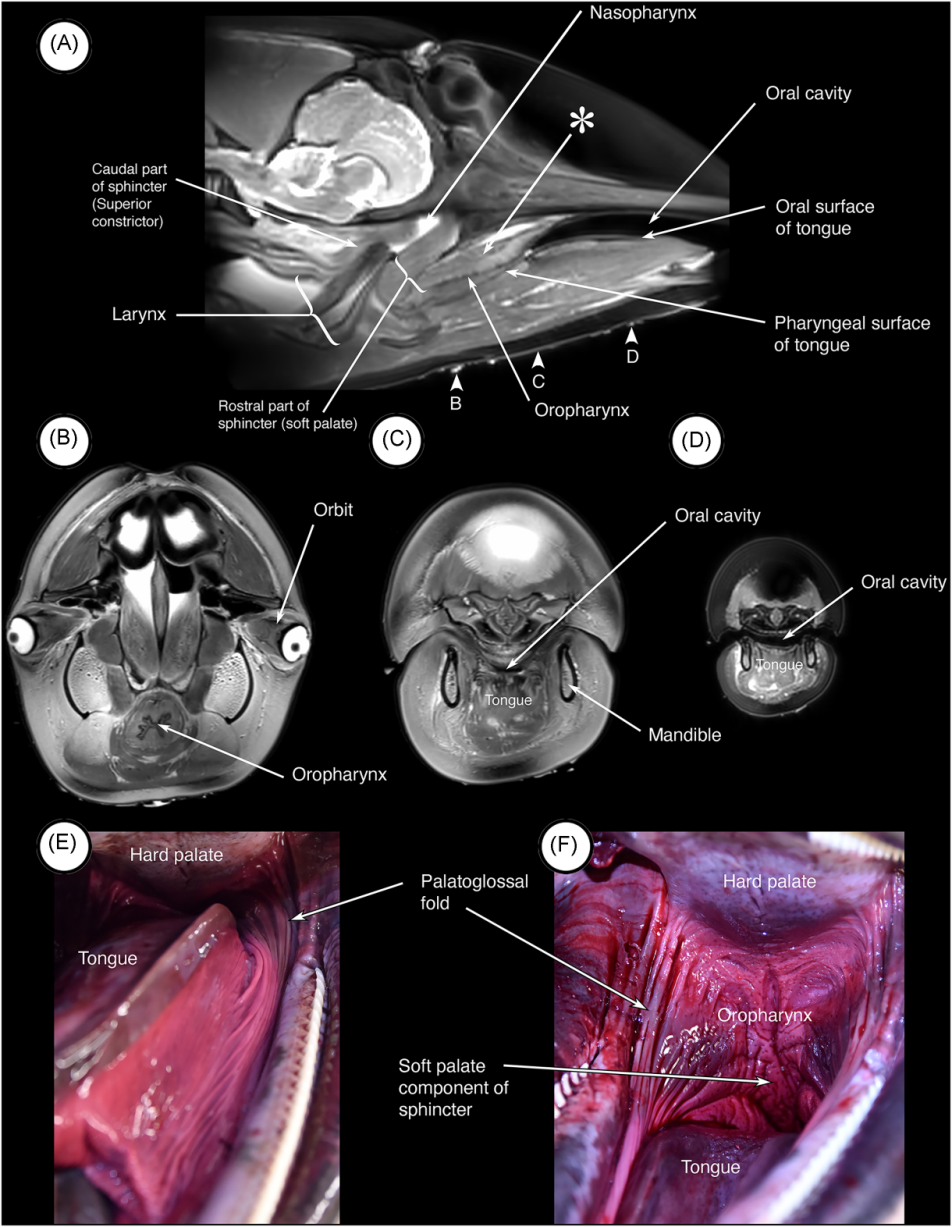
Compartments and landmarks in MRI images and dissection of a pacific white sided dolphin (*A. obliquidens)*. (A) MRI scan in the mid-sagittal plane showing the relevant structures and compartments in the head and neck. The thickened roof of the oropharynx is indicated by the arrow labelled with the asterisk. The arrowheads labelled B, C, and D indicate the positions of the MRI scans in the transverse plane shown in (B), (C) and (D). (E & F) Shown in these images are the palatoglossal folds that mark the lateral margins of the oropharyngeal isthmus.
